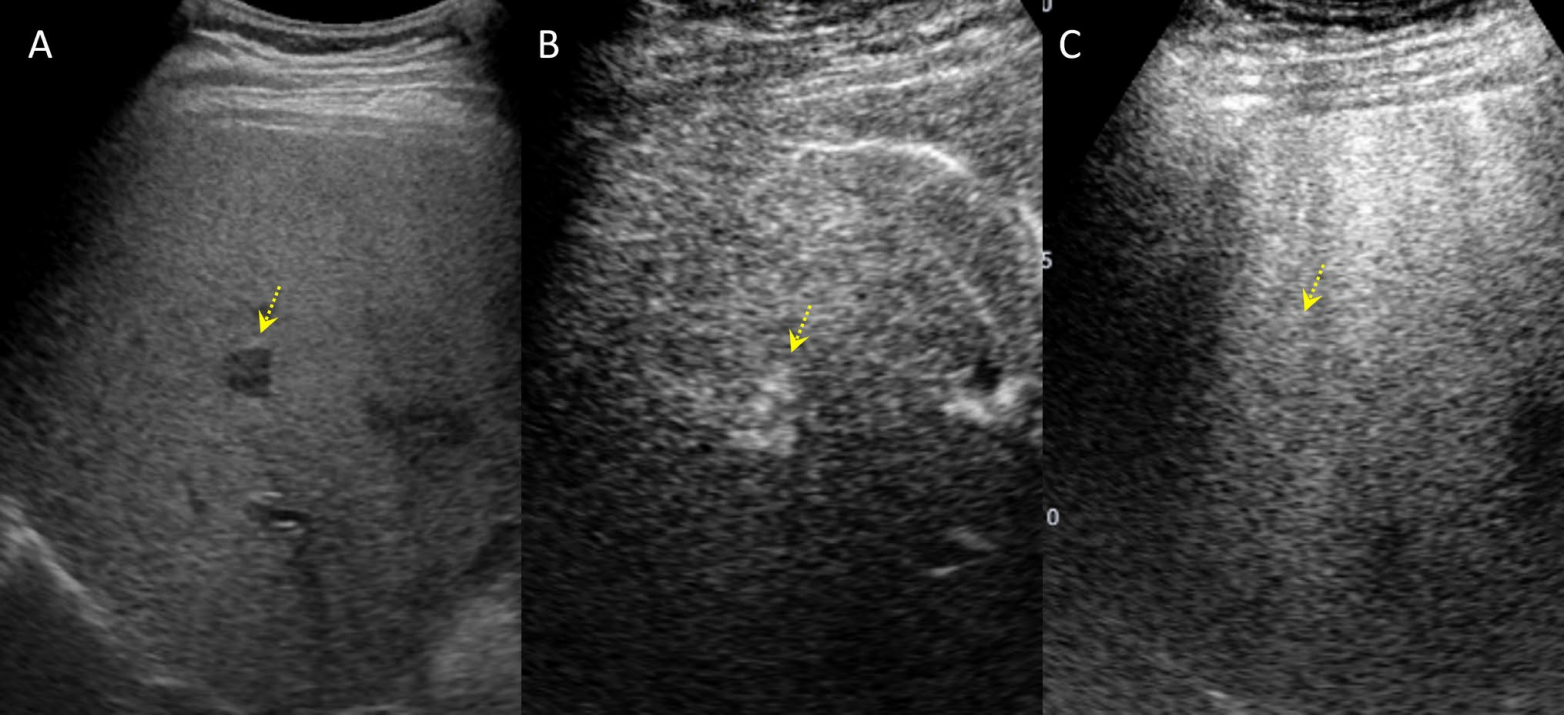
Contrast-enhanced ultrasound images of focal nodular hyperplasia (S8) (dotted yellow arrows) two years after liver transplantation. A The liver nodule was depicted as a 13 mm hypoechoic area in B-mode. B The liver nodule was hyperattenuating in the arterial phase. C There was no defect in the Kupffer phase
